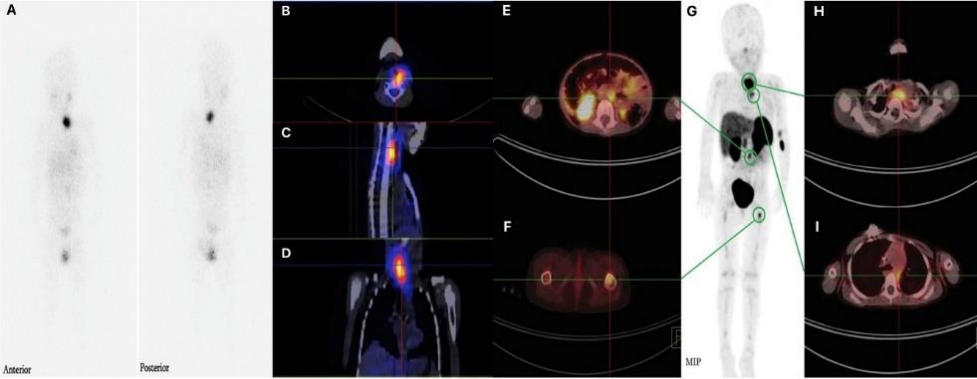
^131^I-MIBG and ^68^Ga-DOTATATE images of a 3-year-old boy with neuroblastoma, taken less than 1 month apart. (A) ^131^I-MIBG whole-body scintigraphic images, together with (B) transverse, (C) sagittal, and (D) coronal SPECT/CT images show a metastatic lesion in the cervical paravertebral region. (G) Maximal intensity projection ^68^Ga-DOTATATE PET image, along with ^68^Ga-DOTATATE PET/CT fusion images (E, F, H, I) depict metastatic lesions in the cervical and mediastinal paravertebral regions, retroperitoneum, and proximal part of the left femur. The lesions demonstrated on ^68^Ga-DOTATATE images clearly outnumber ^131^I-MIBG images
